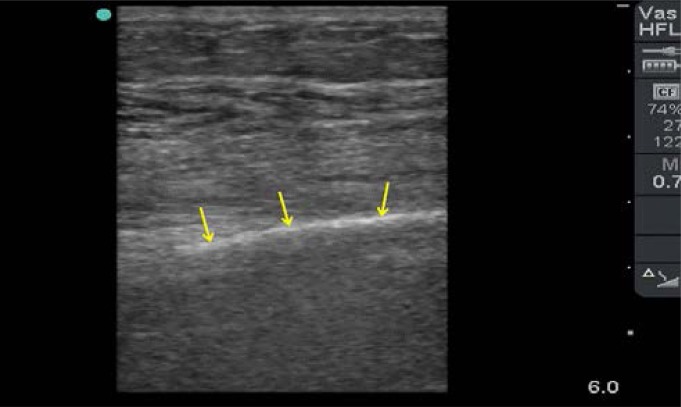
Ultrasound appearance of significant intra-peritoneal free air using a linear probe (10–12 MHZ). There is a hyper-echogenic line (yellow arrows) just under the abdominal fascia at the midline. It does not move with respiration but moves with repositioning the patient. It completely hides the organs deep to it (Courtesy of Professor Fikri Abu-Zidan, Department of Surgery, College of Medicine and Health Sciences, UAE University, United Arab Emirates).
